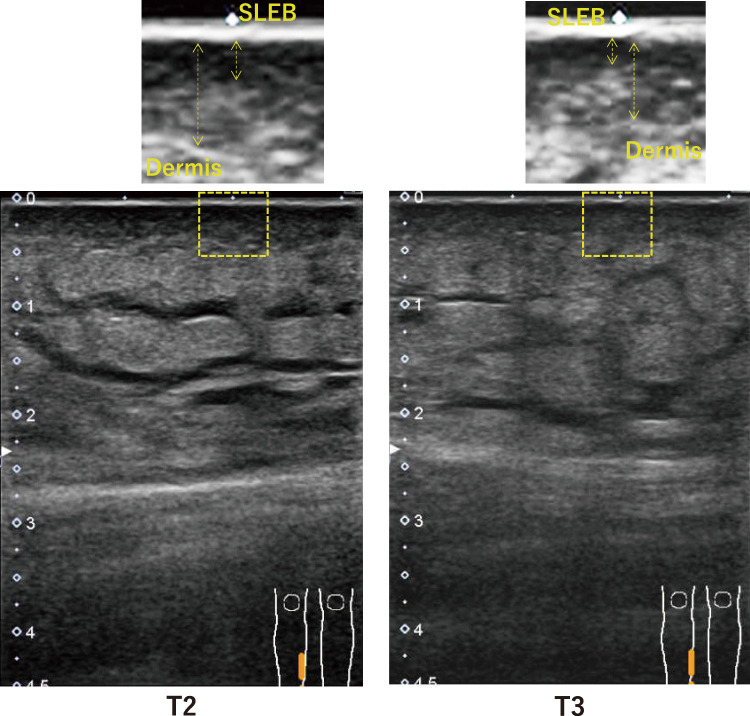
Fig. 3 Representative ultrasonography images of the medial lower calf of a patient in group 2 before (T2) and after (T3) the use of Airbo·Wave EV1. Both dermal and SLEB thickness are reduced after the use of Airbo·Wave EV1. Note that a significant amount of subcutaneous echo-free space, i.e., subcutaneous edema, remains even after the use of Airbo·Wave EV1. SLEB: subepidermal low-echogenic band
